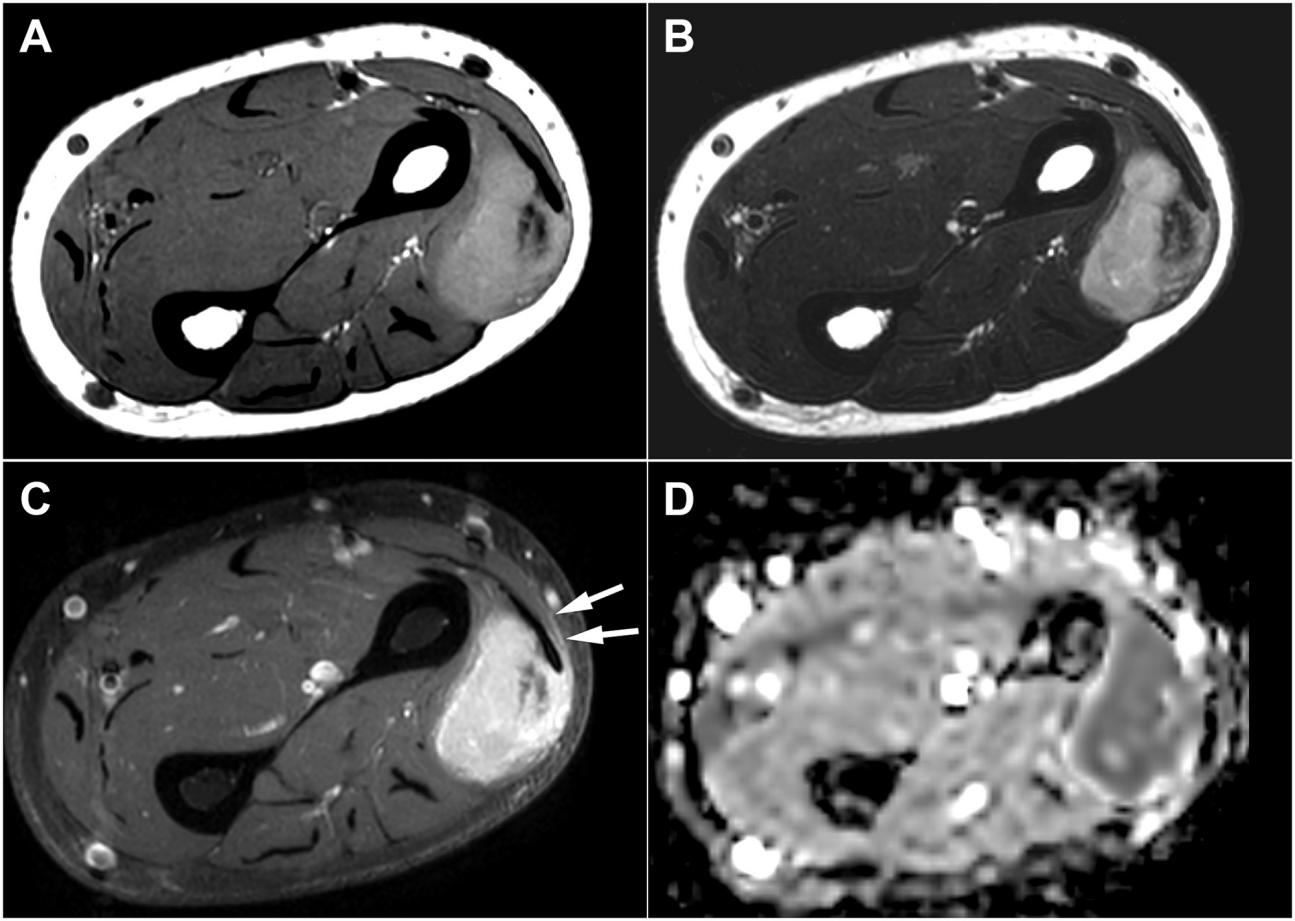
An 80-year-old woman with epithelioid sarcoma. (A) Axial T1- and (B) T2-weighted images of the left forearm showing a 2.7-cm deeply located mass with lobulated contour involving the posterior compartment muscle. (C) Axial fat-suppressed contrast-enhanced T1-weighted image revealed heterogeneous enhancement and the tail sign (arrows). (D) ADC value of the lesion was measured as 1.10 x 10–3 mm2/s. ADC, apparent diffusion coefficient.
